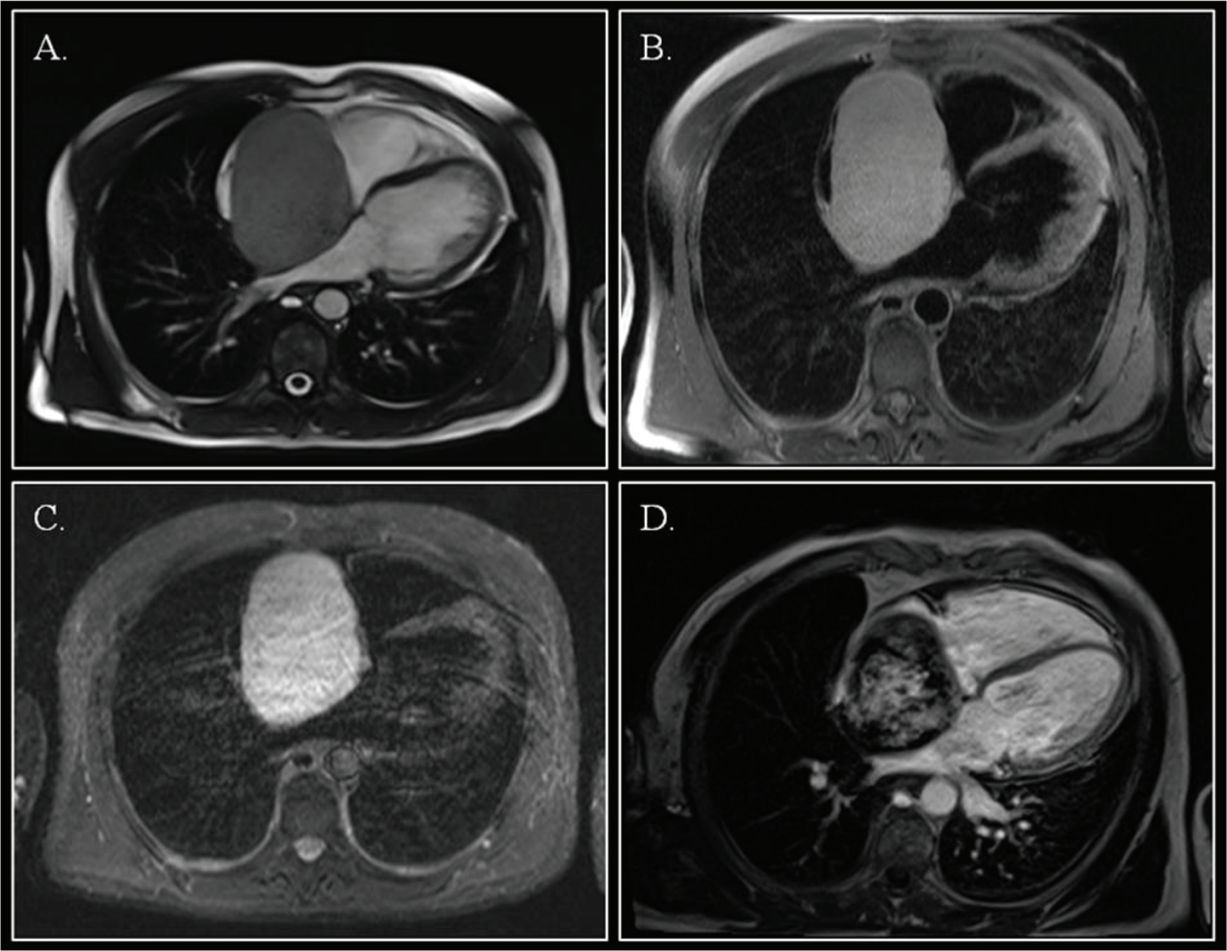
Cardiac magnetic resonance Steady-state free precession cine imaging demonstrated a right atrial mass with a large base of implant on the inter-atrial septum. (Panel A.; still frame, off-axis four-chamber view). The mass was hyperintense on T1 images with fat saturation (Panel B.), and on T2-weighted STIR images (Panel C.). After gadolinium injection, inhomogeneous late gadolinium enhancement was evident (Panel D.).
